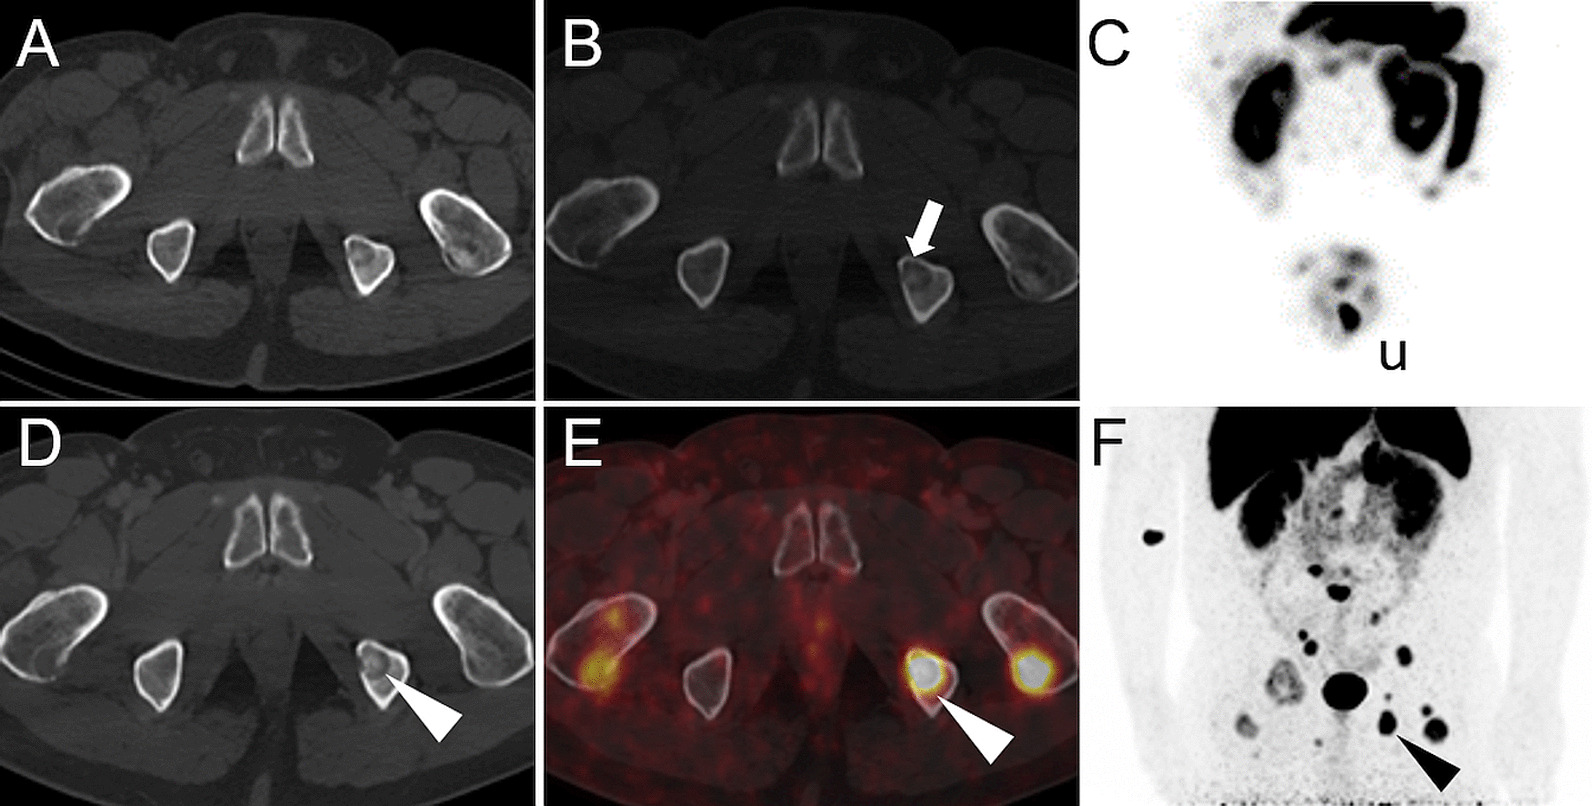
Discrepancy of [^177^Lu]Lu-PP-F11N and [^68^Ga]Ga-DOTATOC uptake in patient 5. Axial CT (A, D), SPECT/CT (B), PET/CT (E) and MIP (C, F) of patient 5. No visible uptake of [^177^Lu]Lu-PP-F11N in SPECT/CT (white arrow, B, with Entresto^®^ premedication) and MIP (C). Intense uptake of [^68^Ga]Ga-DOTATOC in multiple lytic bone metastases (white and black arrowheads, E, F. u: urine contamination
